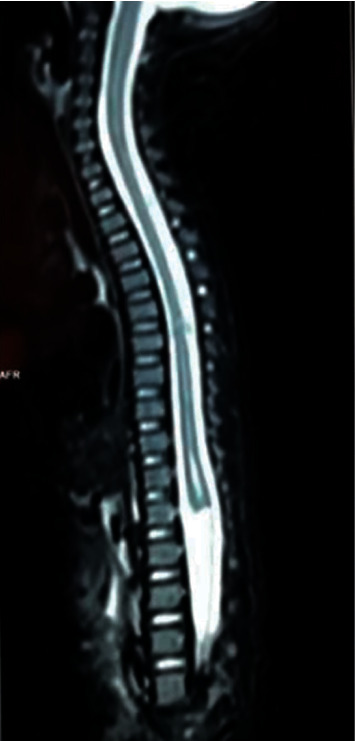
MRI spine: abrupt termination of spinal cord at the L1 vertebra level, syrinx involving D11-L1 measuring 17 mm in length and 1 mm in diameter.
